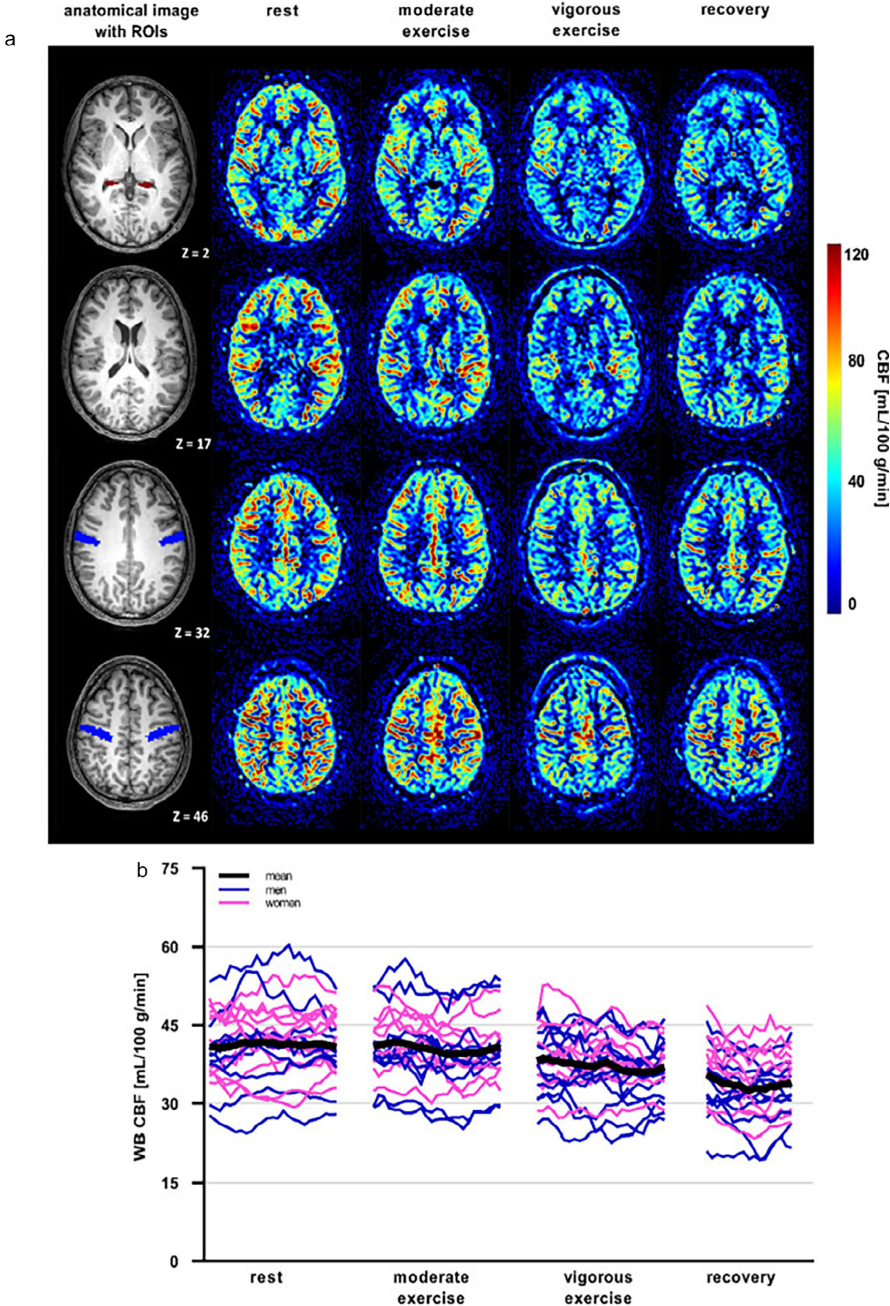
Quantitative maps of cerebral blood flow (CBF) obtained with pseudo‐continuous arterial spin labeling (pCASL) MRI at 3 Tesla at rest, during various stages of steady‐state supine bicycling exercise, and during subsequent recovery (a). Regions of interest for hippocampus and motor cortex are outlined in red and blue, respectively, on T_1_‐weighted anatomical images with the MNI‐space (Montreal Neurological Institute, Montreal, Quebec, Canada) z‐coordinate indicated. Note the enhanced perfusion of the skin during exercise and subsequent recovery. Time curves (temporal resolution, 9.1 s per control/label pair) of the mean whole‐brain (WB) CBF (b) show a consistent decrease during vigorous exercise that continued during recovery. Reproduced from Mast et al^185^ with permission from Elsevier under a Creative Commons BY‐NC‐ND license (https://creativecommons.org/licenses/by‐nc‐nd/4.0/).
